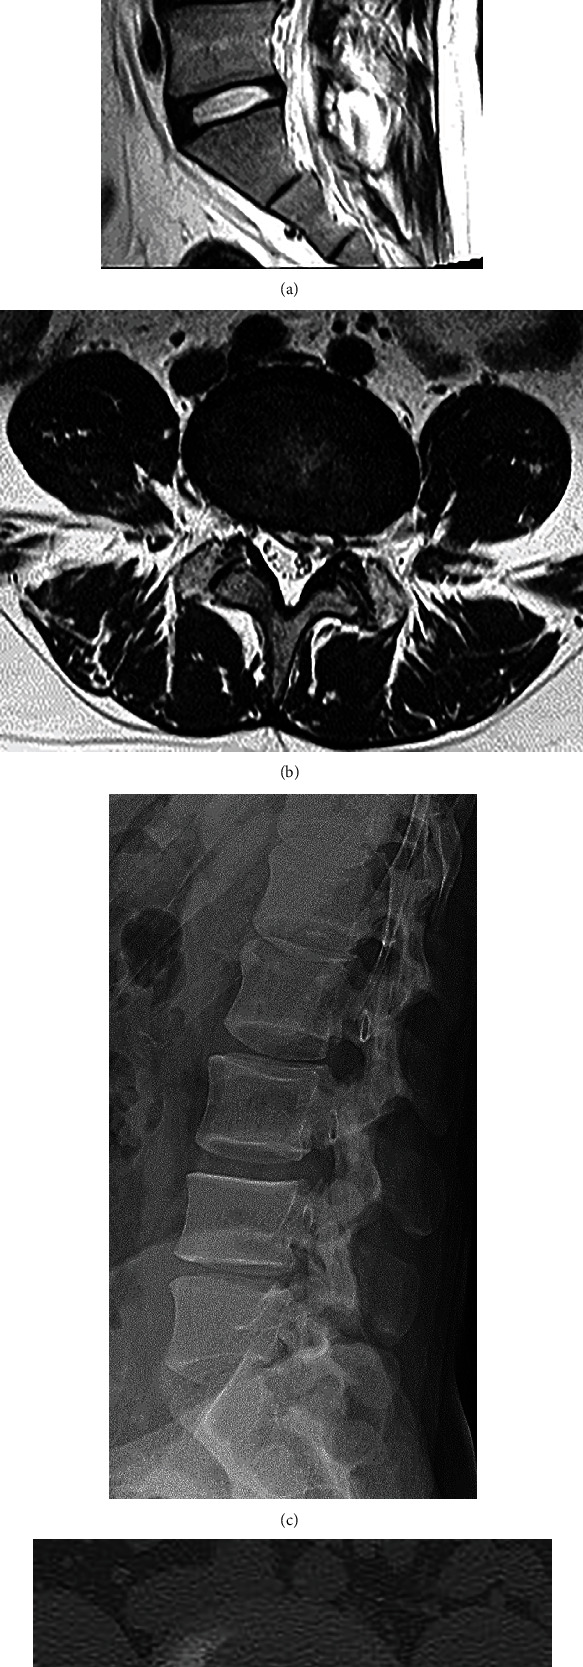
Spinal imaging before intradiscal condoliase injection. A (a) sagittal section and (b) horizontal section of the L4/5 on T2-weighted magnetic resonance imaging showed intracanal, left-sided transligamentous disc herniation with high-signal intensity. (a) The degree of affected disc degeneration was grade III according to the Pfirrmann classification [9]. (c) Standing radiography revealed that the L4/5 disc height was slightly diminished as compared to the other discs. (d) Computed tomography showed no bony stenosis at L4/5.
